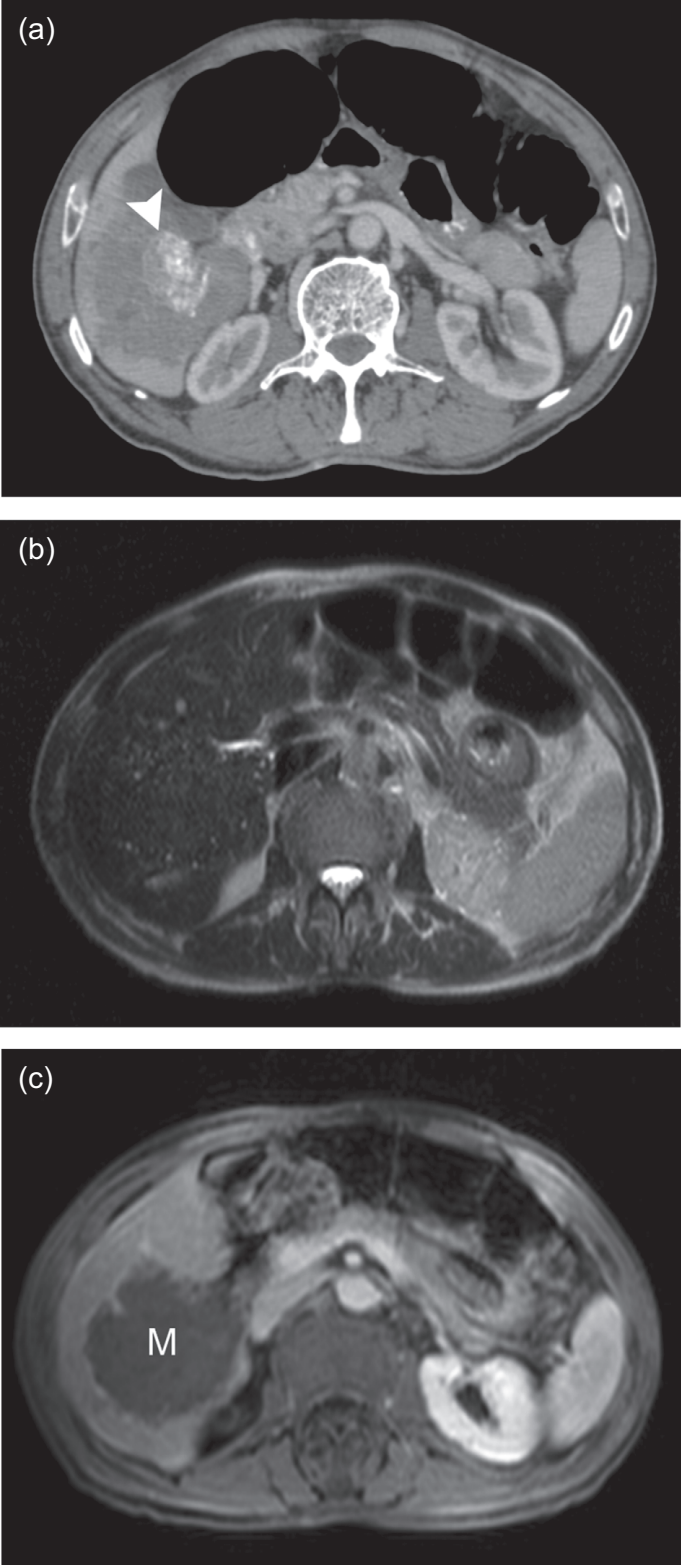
A 78-year-old male patient previously operated for gastric cancer was found to have a mass in the right lobe of the liver. (a) Axial contrast-enhanced CT examination shows an irregularly bordered, hypovascular, heterogeneous mass lesion in the right lobe. Focal calcific areas (*arrowhead*) in the center of the lesion can be clearly discerned. (b) On an axial T2W MRI image, the mass is not clearly discernible from the normal liver parenchyma. However, many hyperintense, small cysts can be seen at the peripheral zones of the lesion. (c) A postcontrast axial MRI image demonstrates that the mass (*M*) did not show prominent contrast uptake with the exception of a weak, peripheral contrast uptake. With an elevated CA 19-9 level, the patient underwent core biopsy to exclude a malignancy, and the result indicated the diagnosis of AE.
